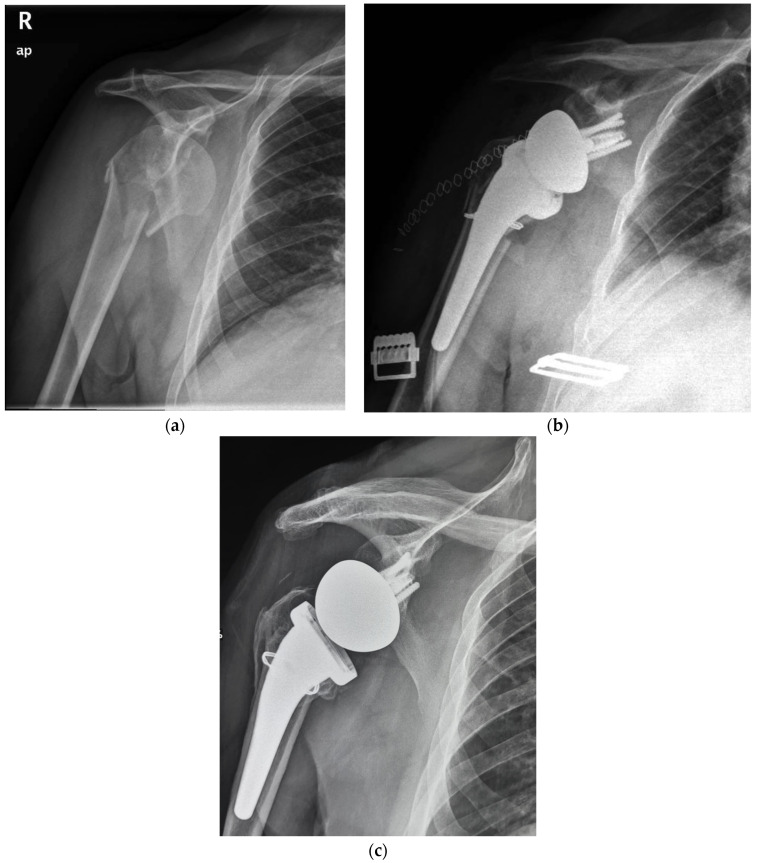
(a) Preoperative radiographic image of right shoulder showing acute (Neer three-part) proximal humeral fracture with calcar involvement. (b) Immediate postoperative film of right shoulder showing calcar fragment fixation with steel wire cerclage. (c) 5.7 years postoperative film of right shoulder showing stem subsidence and healing of tuberosity and medial calcar.
